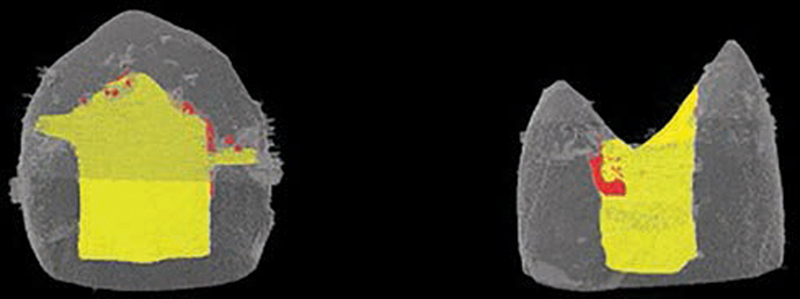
Microcomputed tomography images for microleakage analysis.
